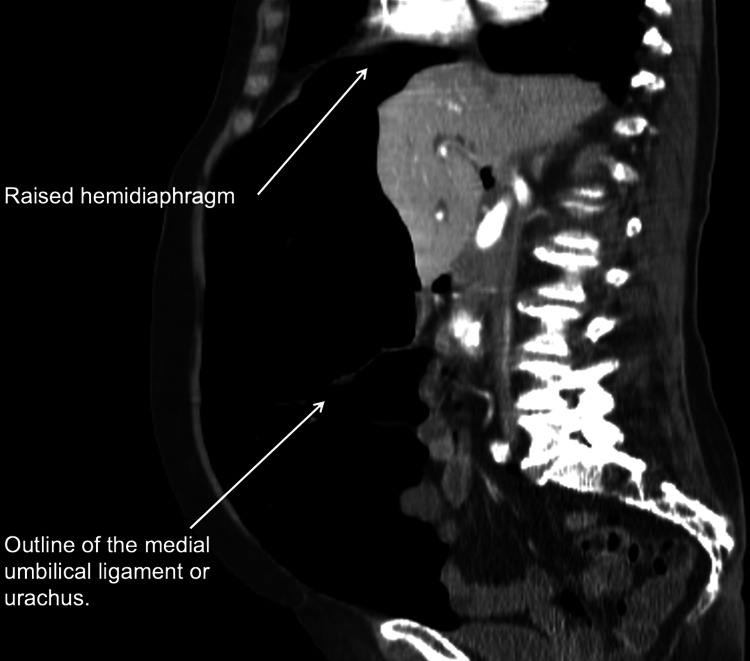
Sagittal CT scan image showing raised hemidiaphragm and the outline of the urachus. CT abdomen and pelvis with contrast: A massive pneumoperitoneum is seen, site of perforation could not be ascertained, no bowel wall thickening, and the other abdominal viscera are unremarkable.
